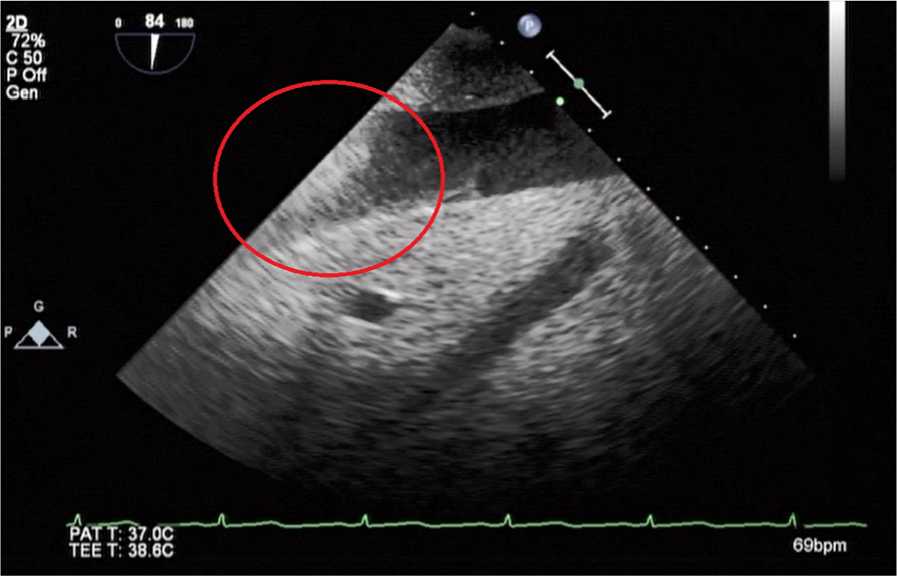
Intraoperative transthoracic echocardiography. Intraoperative transthoracic echocardiography image of the modified transgastric hepatic vein view revealing that the inferior vena cava was almost fully occluded (circle) by a tumor embolus at the level of the inferior hepatic vein
